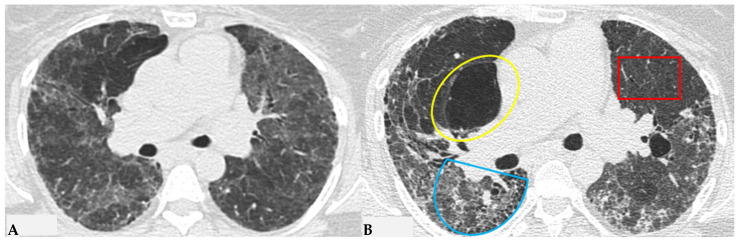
Progressive FHP pattern. Axial chest HRCT scan. Longitudinal assessment of a rapidly progressive form of HP: evident transitions from a cellular form characterized by GGO/centrilobular fluffy nodules and mosaic attenuation (A) to a fibrotic form (B), characterized by distorted fibrotic GGO, traction bronchiectasis and bronchiolectasis (blue shape), a clear increase in the extensive area of air trapping on the right (yellow circle) and an area of lung spared from the pathological process (red box): a picture of progressive FHP with a “three density pattern”.
